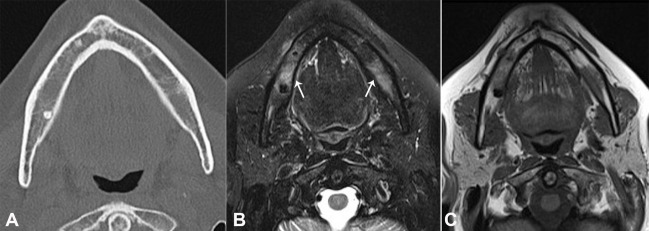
A – Computed tomography of the mandible (axial plane) - note a reduction of bone trabeculations in the mandibular body, possibly related to infiltration; B and C – Axial plane of MRI with bilateral mandibular bone marrow signal alteration (arrows); B – T2 high signal tissue near the right mental foramen related to the involvement of the inferior alveolar nerve (arrow); C – Confirmation of the image in T1-weighted sequence.
